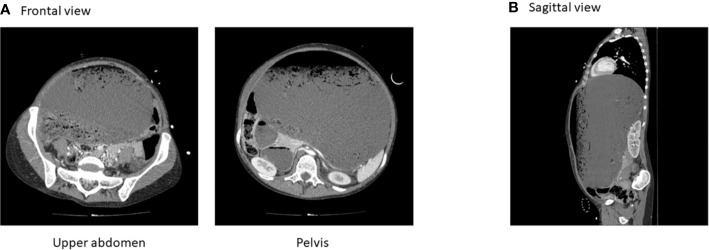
Computed tomography on initial admission. (A) Frontal view. (B) Sagittal view.
